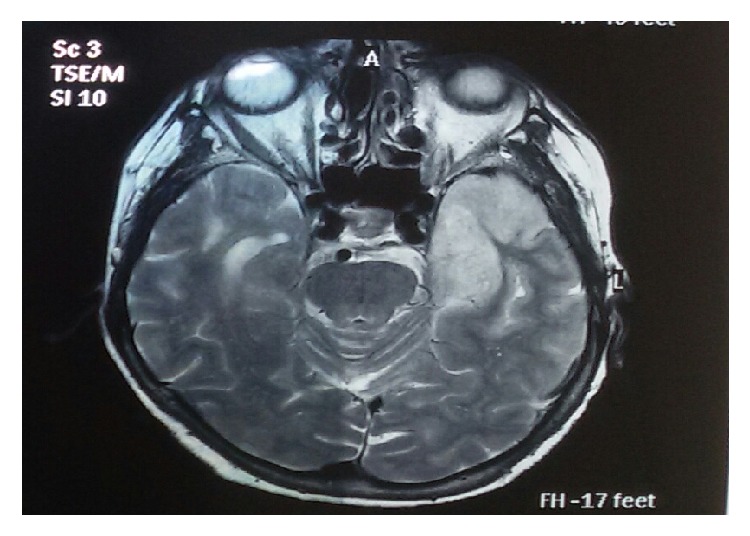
Hyperintense depiction of the left temporal lobe and the insula in T2WI sequence of brain MRI scan, indicative for HSV encephalitis.
